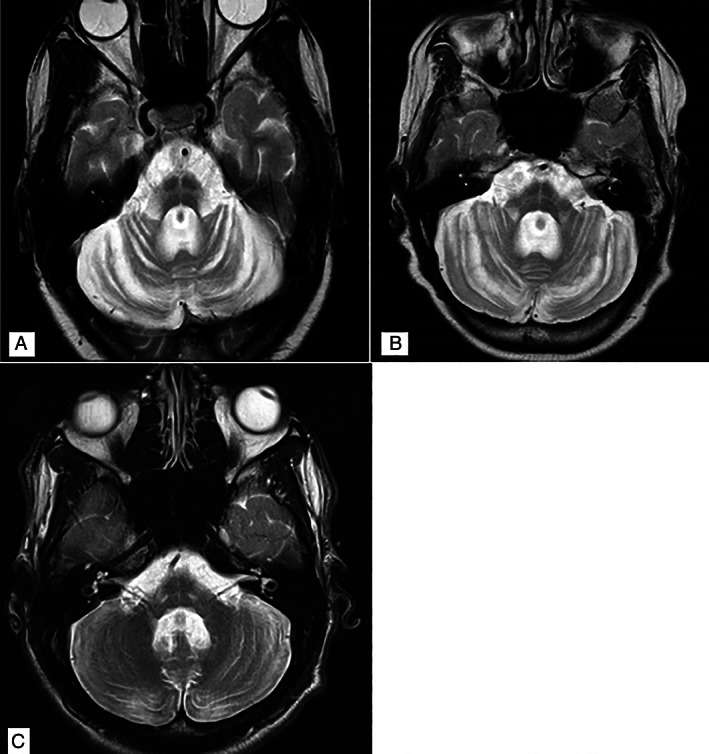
MRI T2W Axial MRI at mid‐pontine level of Case 1 (A), Case 2 (B) and Case 3 (C). A cruciform hyperintensity with atrophy of the brainstem, cerebellum and bilateral MCP hyperintensities are noted in (A, B) and asymmetrically in (C).
